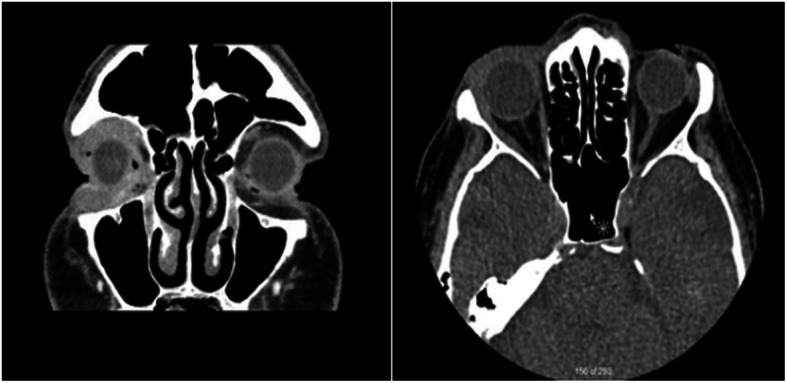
Coronal and axial contrast enhanced CT slices show an ill-defined soft tissue mass anterior to the right globe with extensive infiltration to the pre-septal and post-septal superior and inferior eyelids
